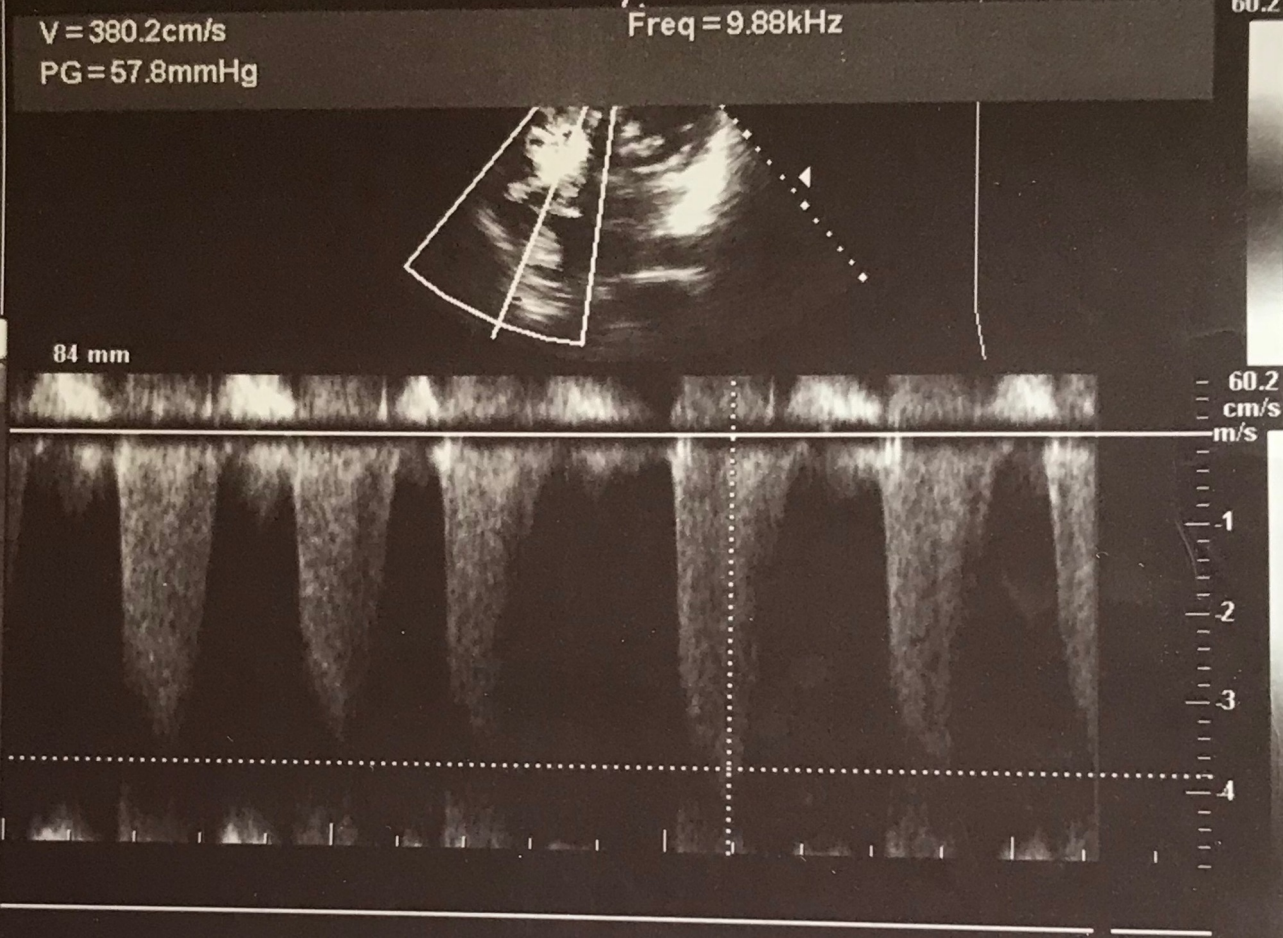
Transthoracic echocardiogram in four-chamber view with continuous wave (CW) mode showing severe tricuspid regurgitation with a pressure gradient of 57.8 mmHg V: velocity; PG: pressure gradient.
